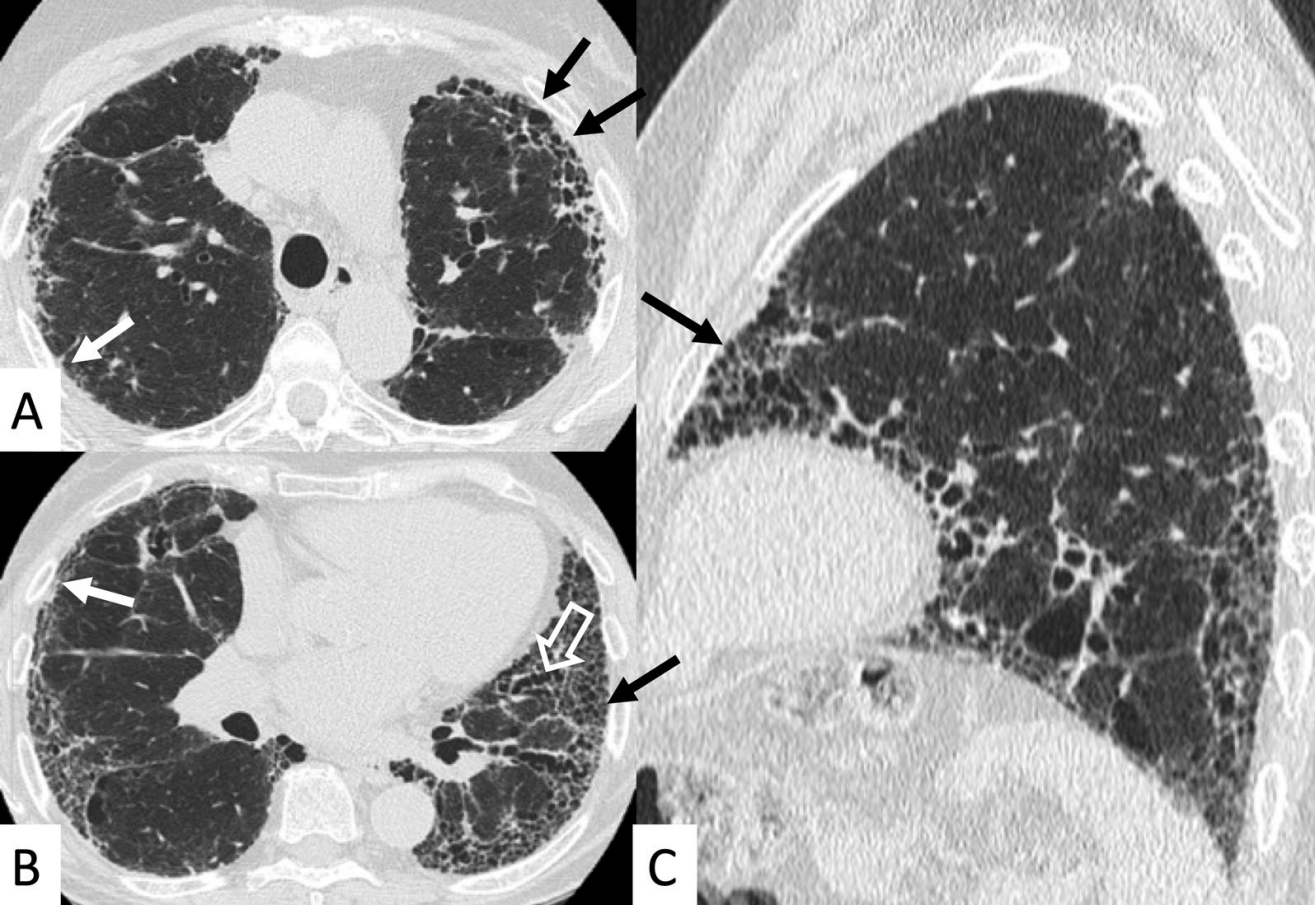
Axial (A, B) and sagittal (C) HRCT images of a UIP pattern in a 72-year-old patient with rheumatoid arthritis. The images show the typical UIP pattern, predominantly located on basal and subpleural regions, and represented by honeycombing areas (black arrows in A–C), reticulations (white arrows in A, B) and traction bronchiectasis (white empty arrow in B)
